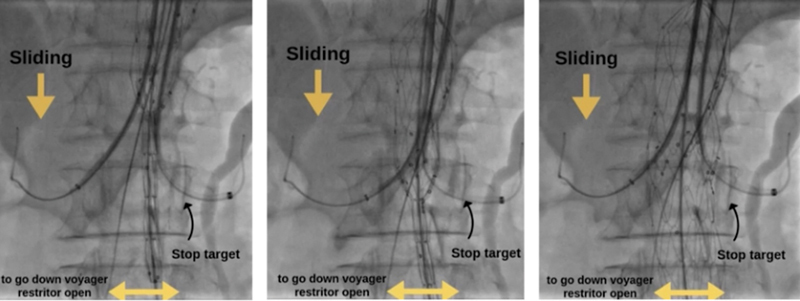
Radiographic representation of the Mandelli sliding rail technique. The image on the right shows us, after performing the maneuver, the release of the endoprosthesis diameter 60% restrictor.
